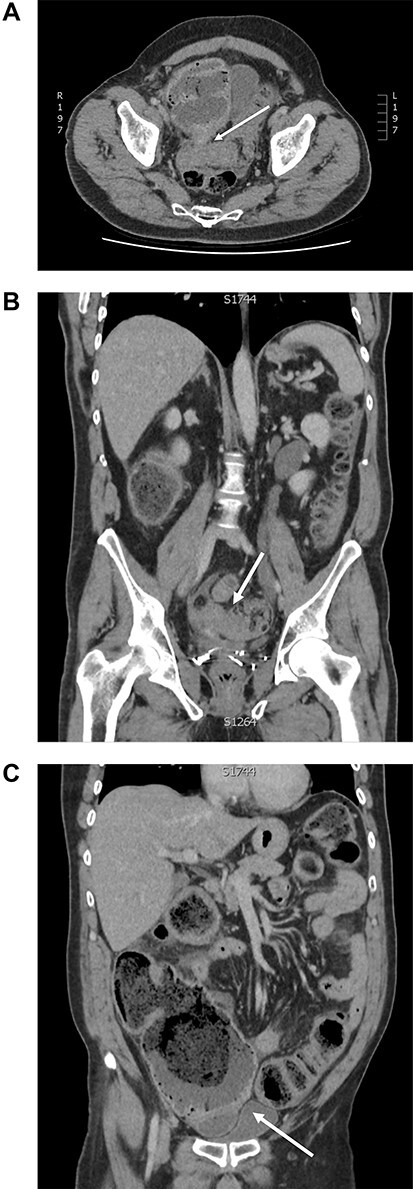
Computed tomography of large bowel obstruction and pneumoperitoneum: (A) axial view depicting dilated caecum with pneumatosis and adherence to the sigmoid stricture (white arrow); (B) coronal view depicting sigmoid stricture (white arrow); (C) coronal view depicting pneumoperitoneum, dilated caecum with adhesion to sigmoid colon stricture (white arrow).
